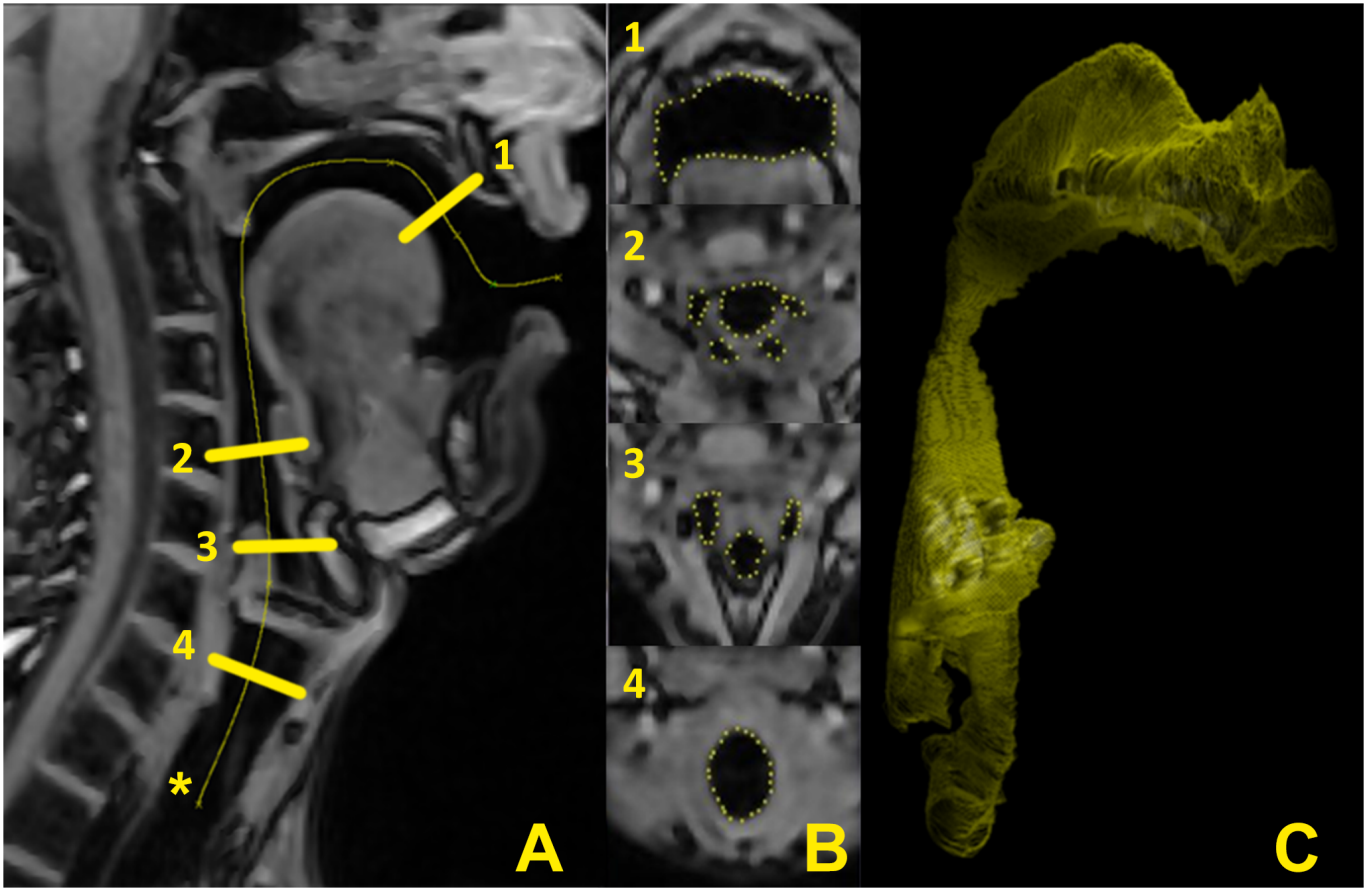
From the MRI to the segmented vocal tract. Typical example of the centerline (*) position used for segmentation (A). Four examples of cross-sections at different levels, as indicated (B). The corresponding 3D representation of the segmented vocal tract (C).
